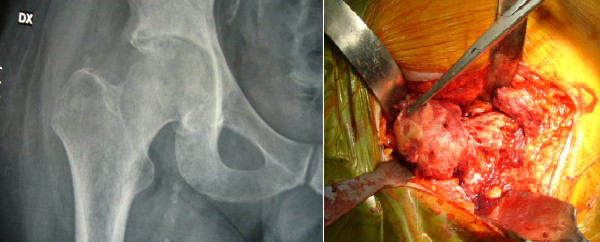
Clinical case (patient GF). Pre-operative X-ray and intraoperative finding. Hematogenous septic hip arthritis.
